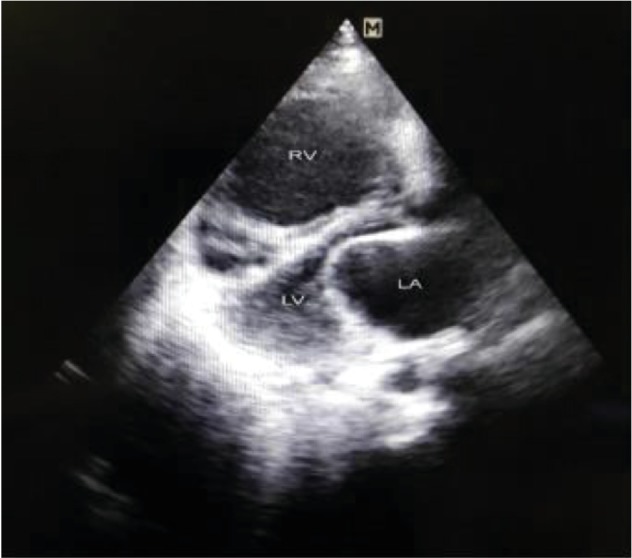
PLAX view 2D echocardiogram picture showing dilated RV and restricted MV opening.
